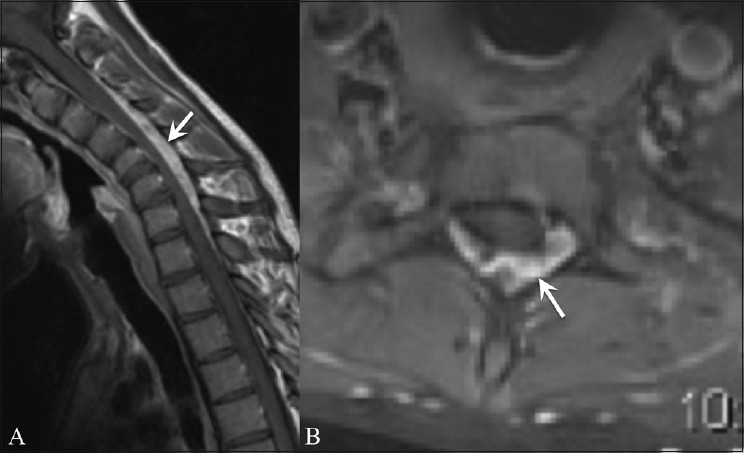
Sagittal flexion (A) and axial (B) contrast-enhanced magnetic resonance images show an enhancing epidural crescent (arrows)
